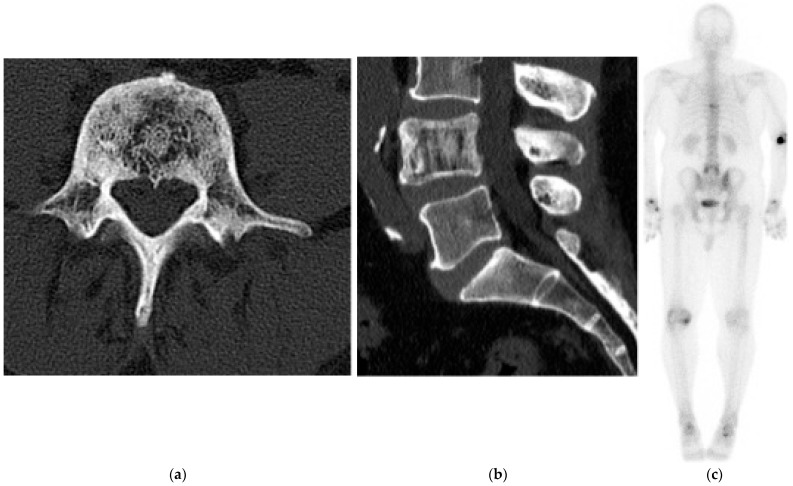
Paget. (a) Axial CT bone window, heterogenous appearance of the vertebral body and posterior elements with areas of lucency and areas of sclerosis with thickening of the trabeculae. (b) Sagittal CT bone window, thickening of the peripheral cortical bone and the central trabeculae. (c) MDP bone scan, corresponding increased uptake in the L4 vertebral body.
